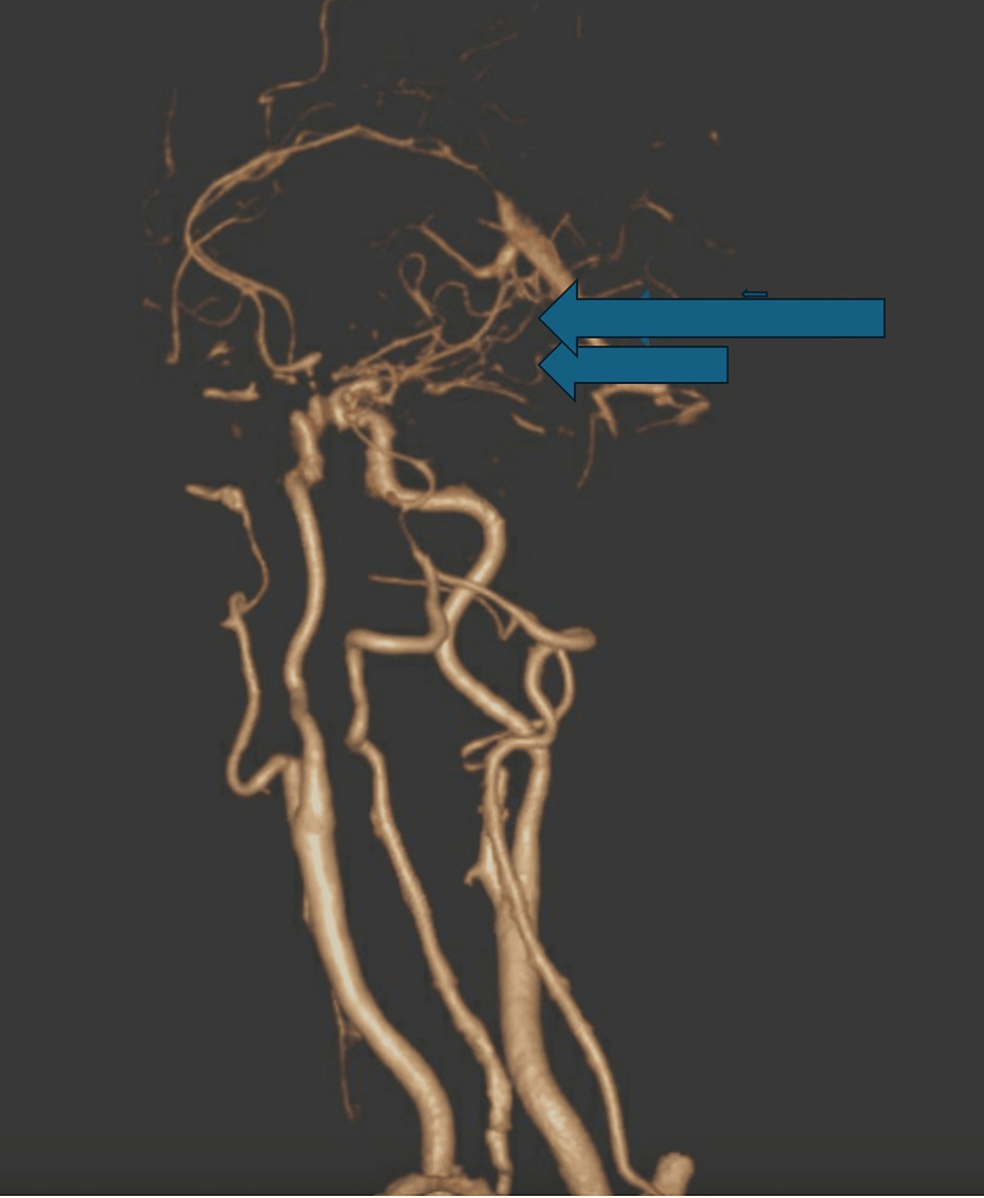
CT angiography showing wispy collateralization characteristic of moyamoya disease (blue arrows).
